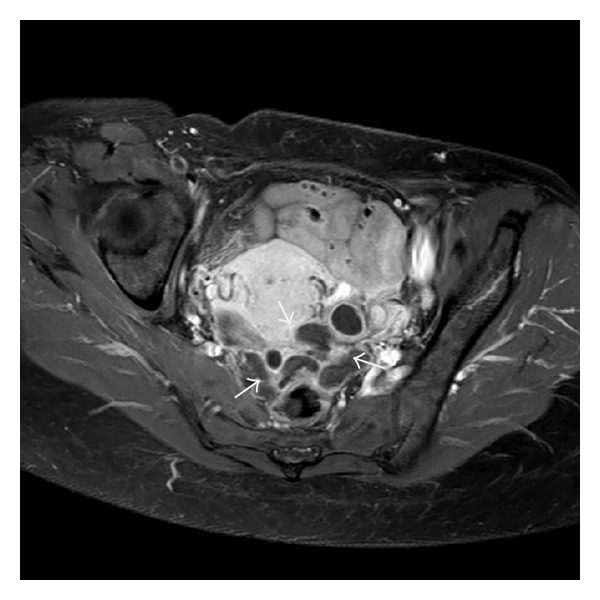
On axial fat-suppressed T1-weighted images after gadolinium administration the masses are strongly hypovascular with a peripheral rim-like enhancement.
